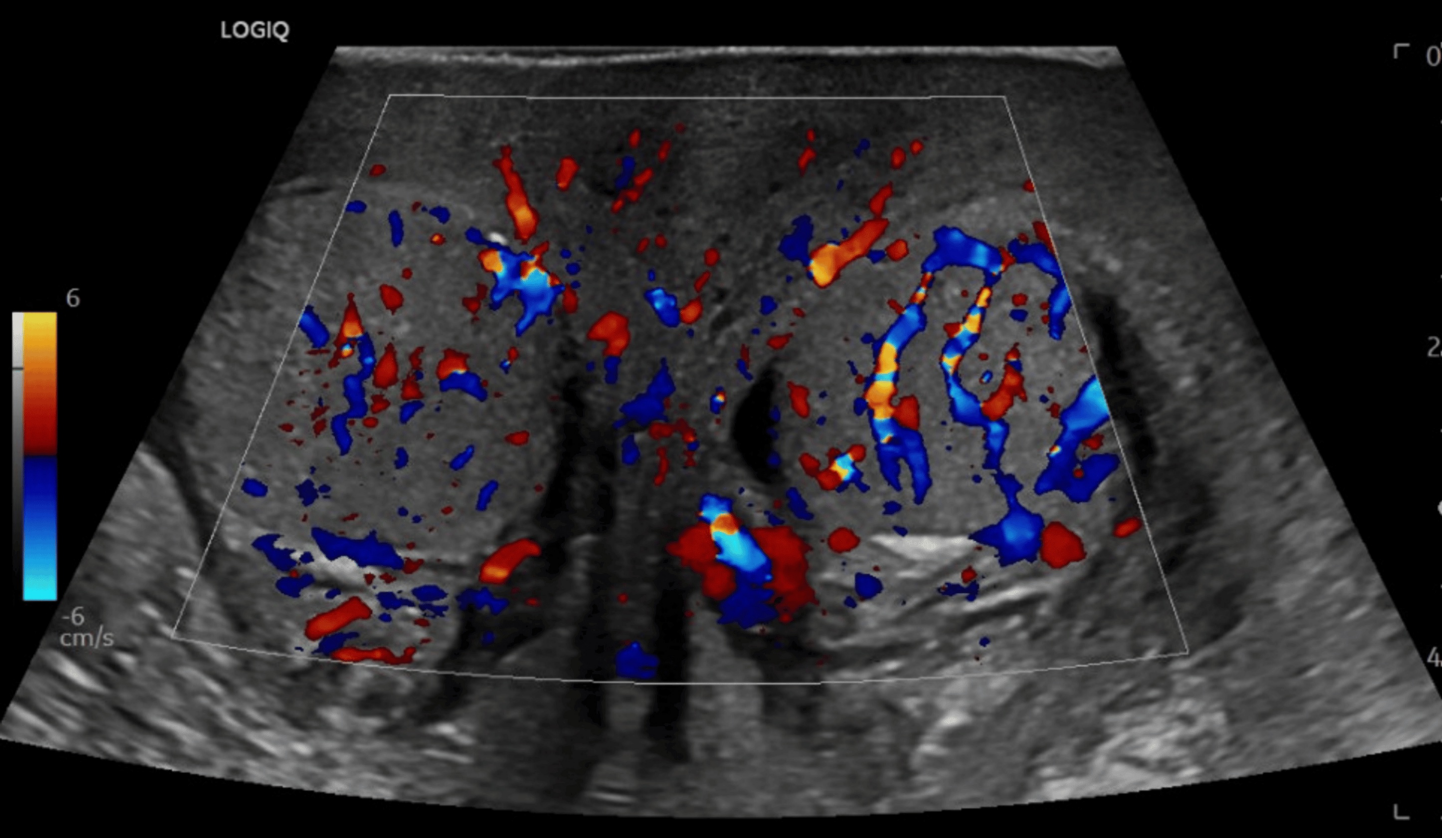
Ultrasonogram of scrotum: slightly hyperemic left testicle without focal lesion, which could be secondary to the scrotal swelling vs orchitis.
